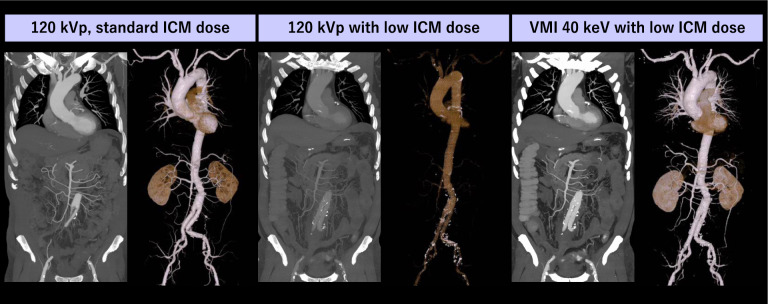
A 78-year-old man with a history of aortic replacement underwent follow-up CT scans at one-year intervals using both standard ICM dose (78 mL) and low ICM dose (25 mL) protocols on single-energy and dual-layer detector CT scanners, respectively. The standard image was reconstructed using conventional 120 kVp, while the low ICM dose images were reconstructed both with conventional 120 kVp and VMI at 40 keV. The reduction in arterial enhancement in the low ICM image was fully compensated by the VMI at 40 keV, resulting in even better arterial depiction compared to the standard ICM dose
